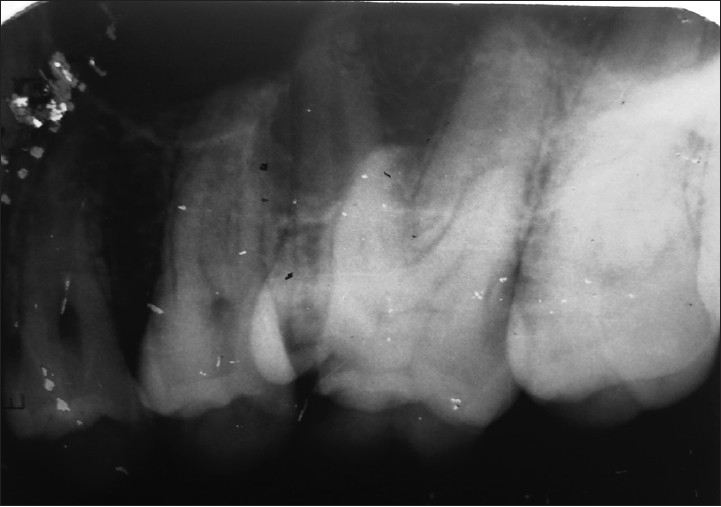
Preoperative radiograph of 26
